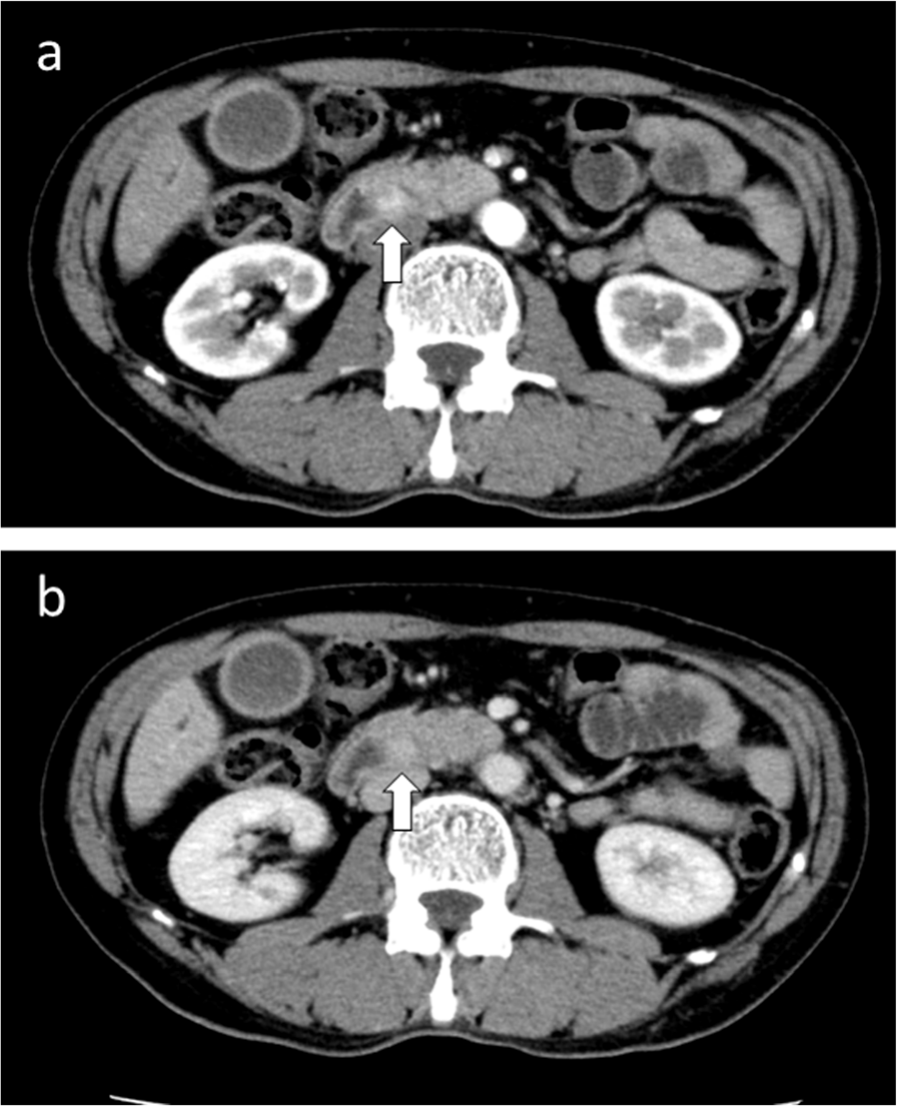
Abdominal contrast-enhanced CT scan image. a The tumor of 11 mm in diameter at the duodenal papilla showed enhancement in the arterial phase (white arrow). b The enhancement of the tumor lasted until the portal phase
